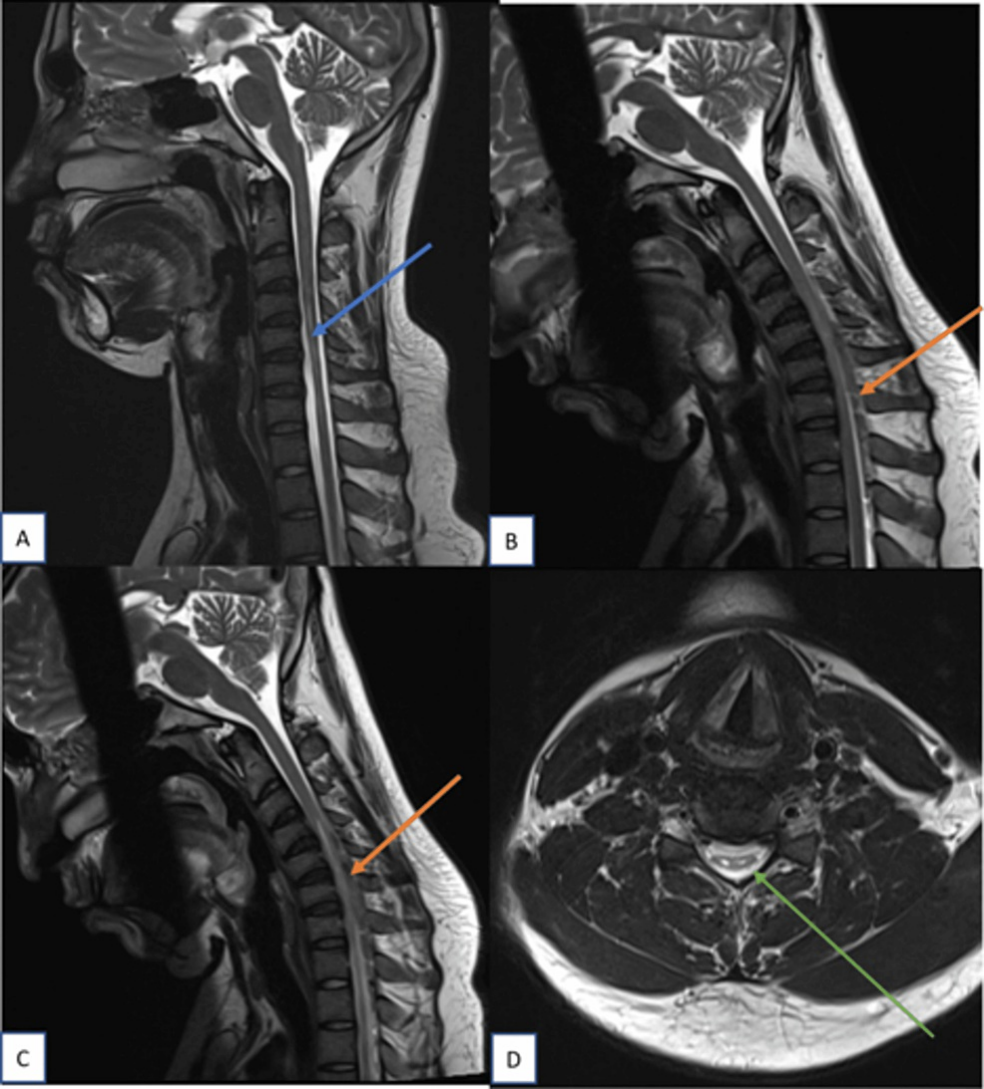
Sagittal T2W images of cervical spine in neutral and flexion position; axial image at the level of C6-C7 intervertebral disc. In neutral position (A), cord atrophy noted from C4  to T1  and T2 hyperintensities from C4  to C7 (blue arrow). In flexion position (B,C), forward displacement of posterior dura (orange arrow) noted from C4 to T3 with maximum displacement at level of C6-C7 intervertebral disc. Axial T2-weighted image (D) at level of C6-C7 intervertebral disc showing asymmetrical flattening of cord and T2 hyperintensities within (green arrow).
